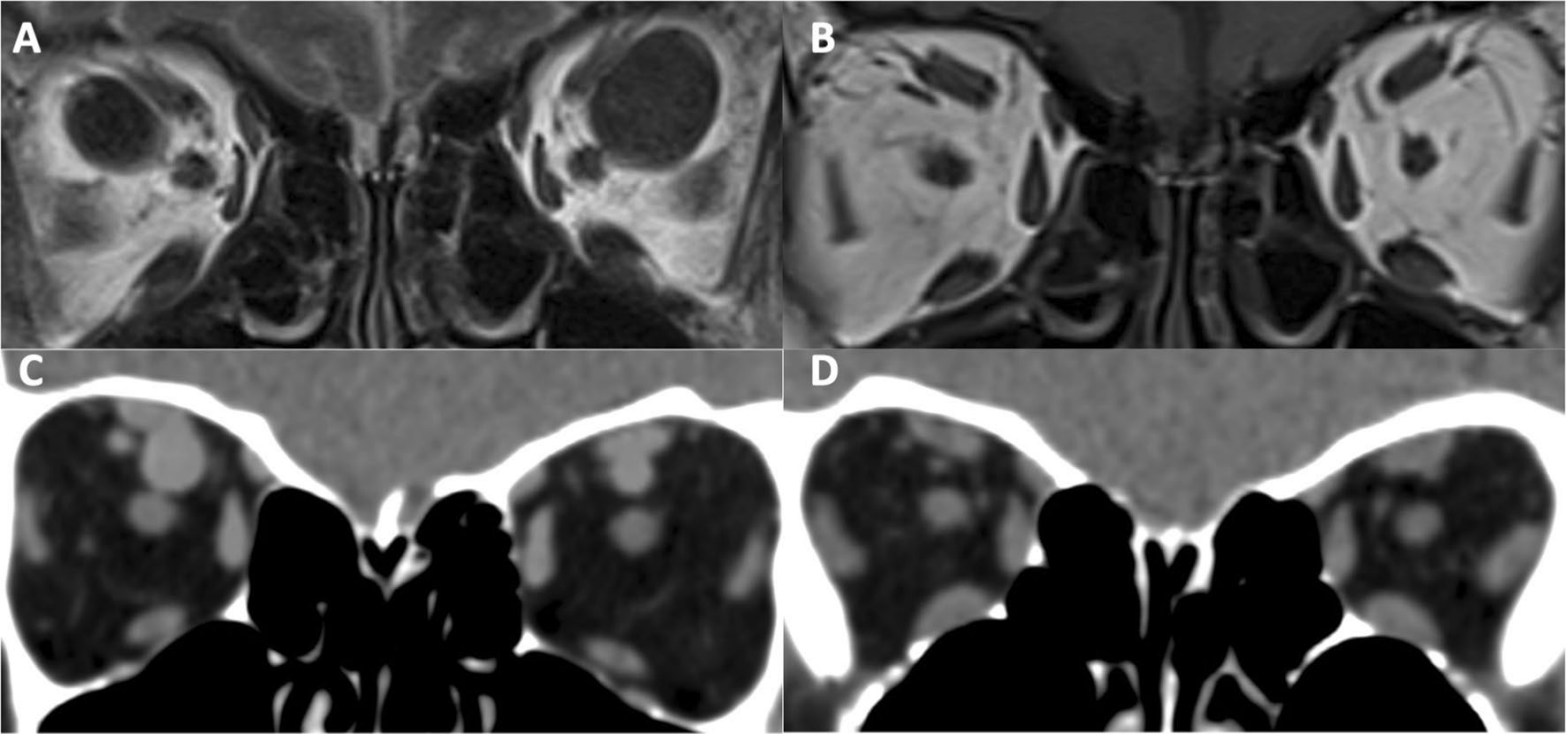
Initial T1-Weighted coronal MRI (A) from Case 1 showing bilateral SOV dilatation. This patient presented with decompensated right heart failure. A follow up scan (B) two years later revealed normal SOVs. Initial coronal CT scan (C) showing bilateral SOV dilatation in Case 7. This patient had a pericardial effusion secondary to breast cancer. A follow up CT scan (D) showed normal SOVs
